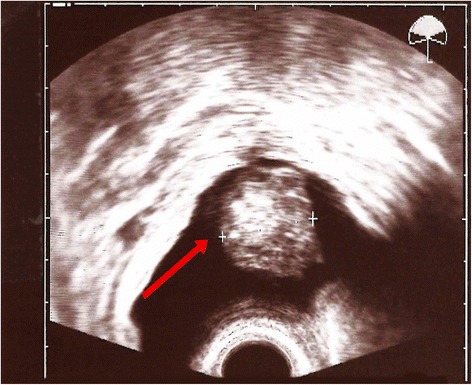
Transvaginal ultrasound of the continent urinary reservoir showing a 3cm calculus. The arrow points to the big calculus in the continent urinary reservoir.
